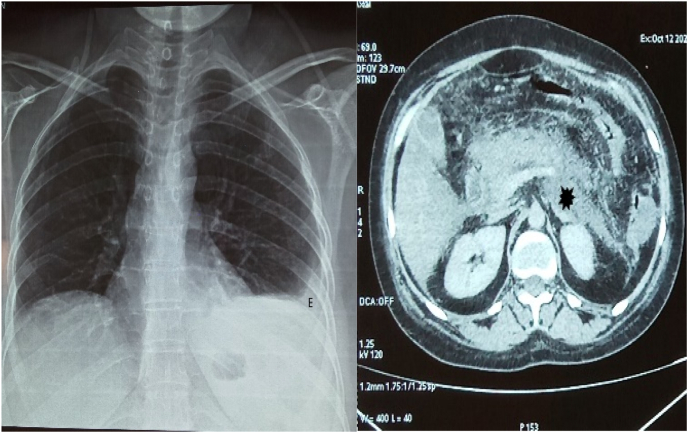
Thoracic X-ray note the pleural effusion (E) and CT scan showing a stage E acute pancreatitis with necrosis (black asterisk).
